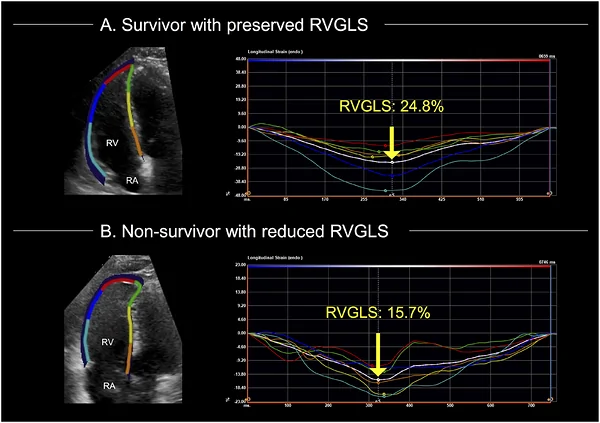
Representative baseline RVGLS in a 30‐day survivor and non‐survivor. RV‐focused apical four‐chamber views with two‐dimensional speckle‐tracking analysis are shown. A representative 30‐day survivor demonstrates preserved RVGLS (24.8%), whereas a representative 30‐day non‐survivor shows reduced RVGLS (15.7%). Strain values are presented as absolute values. RV, right ventricle; RVGLS, right ventricular global longitudinal strain.
